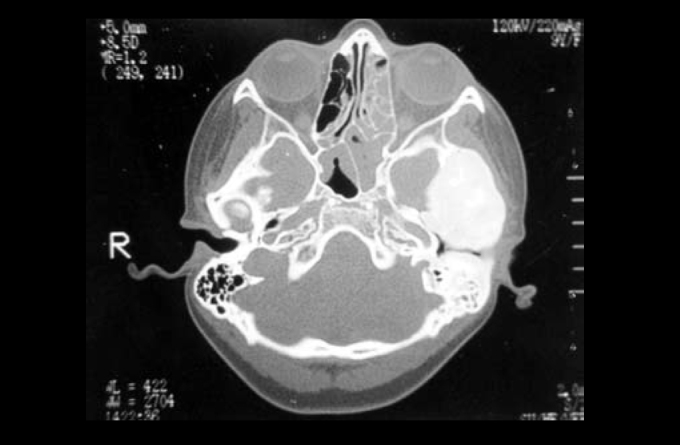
Temporal bone CT scan (axial section) of a patient with fibrous dysplasia of temporal bone on the left, as differential diagnosis. Note the spongiotic bone.
